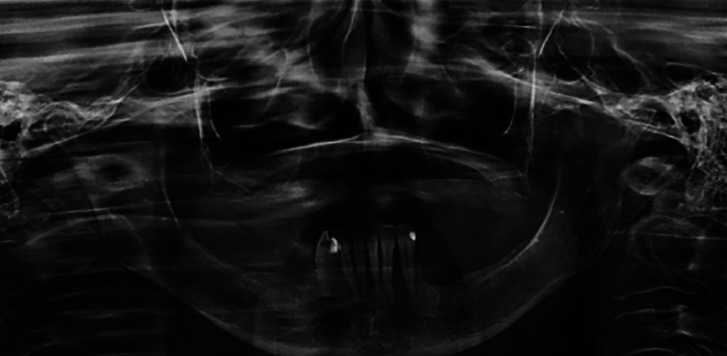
Panoramic radiography after two years of follow-up showing absence of the lesion.
